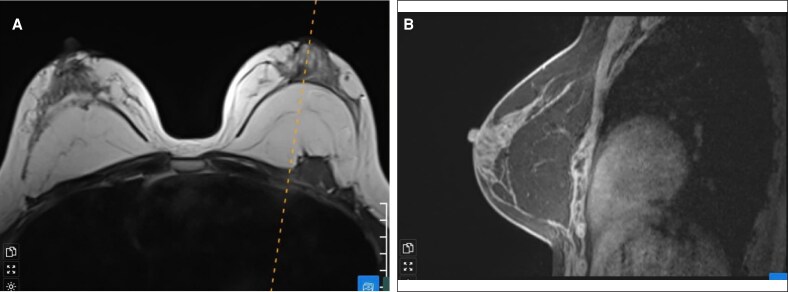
The patient, a 38-year-old woman, underwent explantation because of right-sided implant rupture. (A) Postoperative MRI at 12 months following bilateral implant removal and autologous breast augmentation with transverse rectus abdominis myocutaneous (TRAM) flap and fat grafting. Axial MRI shows well-integrated TRAM flap volume with symmetric fat distribution in both breasts. (B) Postoperative MRI at 12 months following bilateral implant removal and autologous breast augmentation with TRAM flap and fat grafting. Sagittal MRI reveals stable fat graft retention and soft-tissue volume without evidence of oil cysts, seroma, or fat necrosis.
